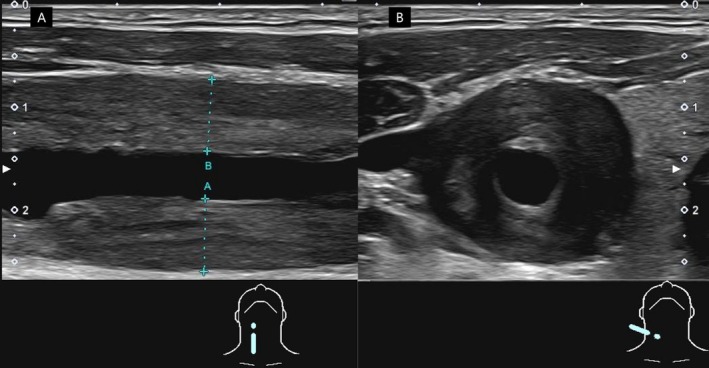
Carotid ultrasonography of the right common carotid artery. (A) Longitudinal view reveals smooth, homogeneous wall thickening. (B) Transverse view reveals concentric circumferential thickening, consistent with the “macaroni sign”.
